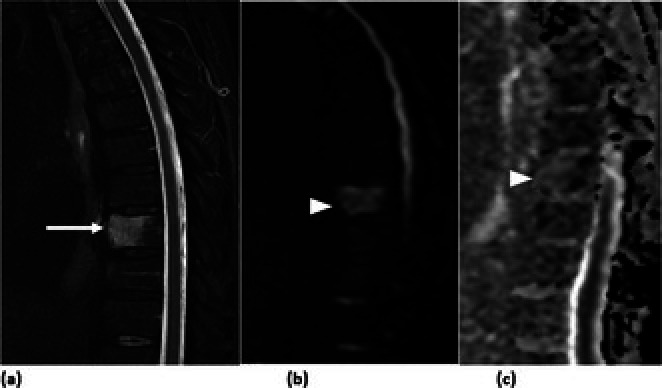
FIGURE 3 T2 STIR (a) image of dorsal spine show abnormal signal intensity (arrow) along the D8 vertebral body with DWI (b) hyperintensity (arrowhead) and ADC (c) image showing partial signal drop suggesting partial diffusion restriction.
